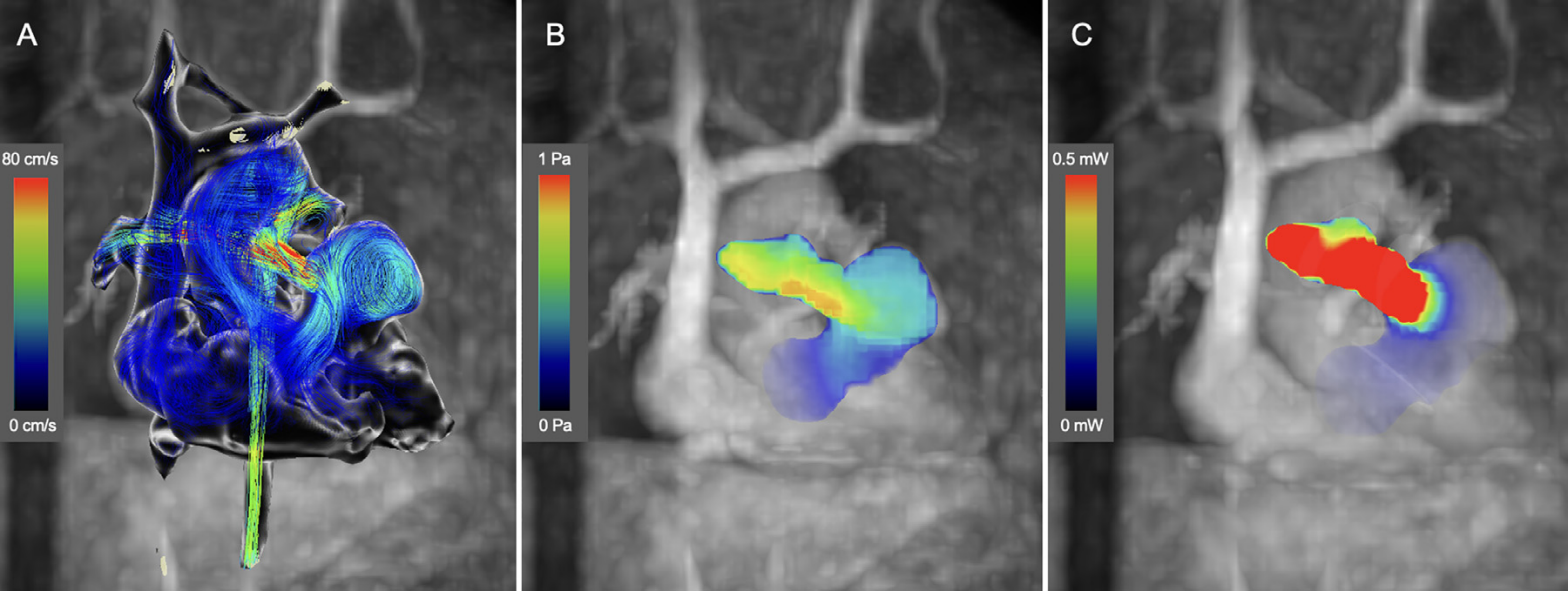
(A-C) Preinterventional 4D Flow MRI. (A) The streamline shows marked vortex flow at the aneurysm of the RVOT in the systolic phase. (B) A peak WSS of 0.18 Pa and an average WSS of 0.09 Pa. (C) A peak EL of 0.01 mW and an average EL of 0.01 mW within the RVOT aneurysm.
